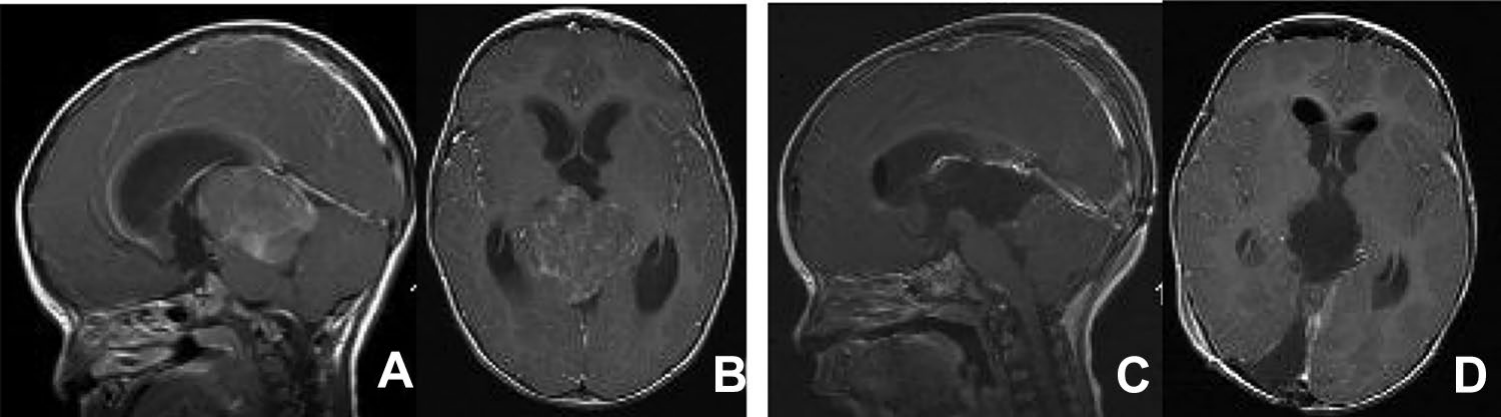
Post-contrast MR, sagittal (A), and axial (B) images show an enhancing large JPA extending from the posterior third ventricle to the bilateral thalamus. The tegmentum and tectum of the midbrain are obscure. Postresection of JPA, MRI, sagittal (C), and axial (D) images showing resolution of the tumor mass and re-appearance of the compressed midbrain. The tumor appeared to arise from the right posterior thalamus extending to the third ventricle
